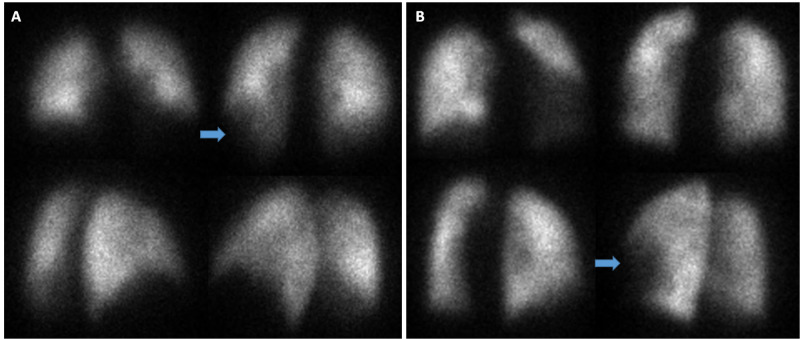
Perfusion scintigraphy for Patients A and B. Although segmental defects are indicated with arrows, the key take-home point or revelation lies in the overall *perfusion pattern *being one that is *heterogeneous *and not homogeneous. This reflects an abnormal state that is multi-lobular and one that is posited to reflect compromised perfusion in this hypoxic condition.
